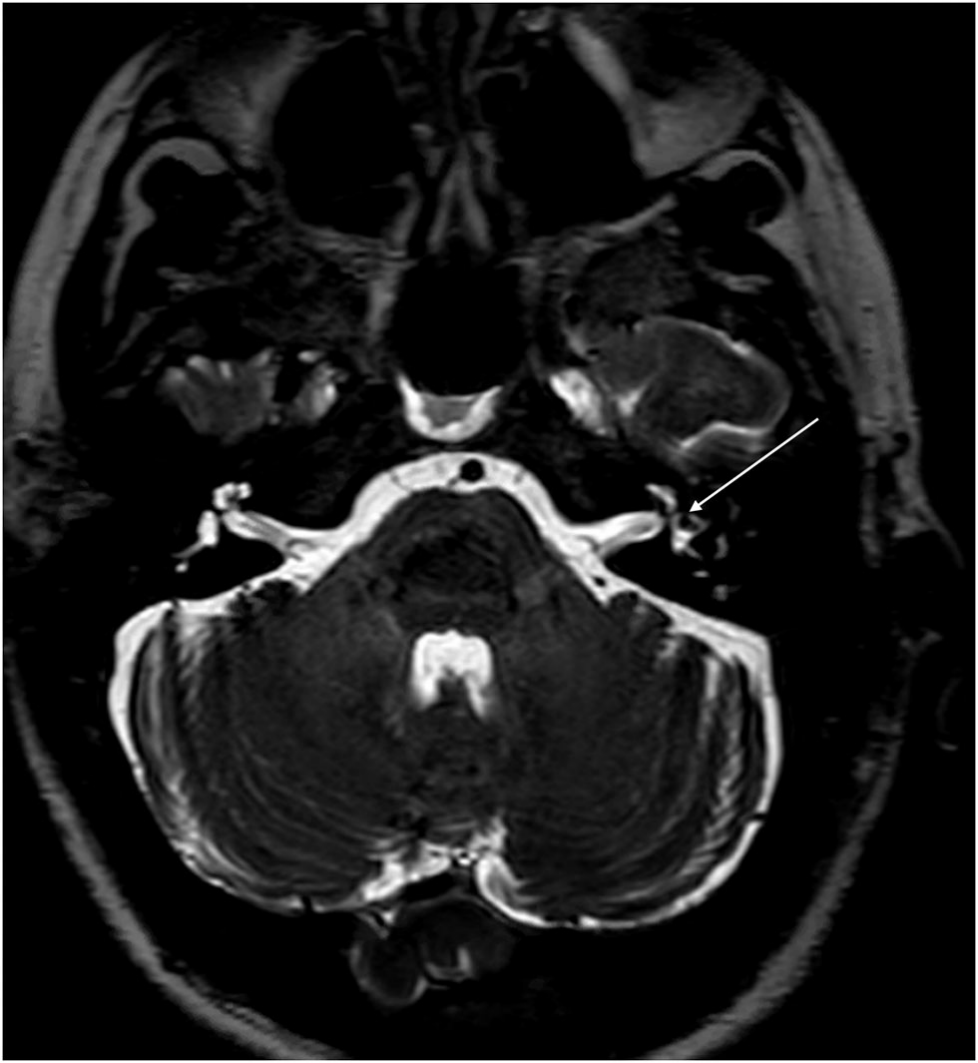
CISS sequence MRI of the same patient as Figure 6 at the level of the vestibule demonstrating improvement in the intravestibular air (arrows) compared to Figure 5.
